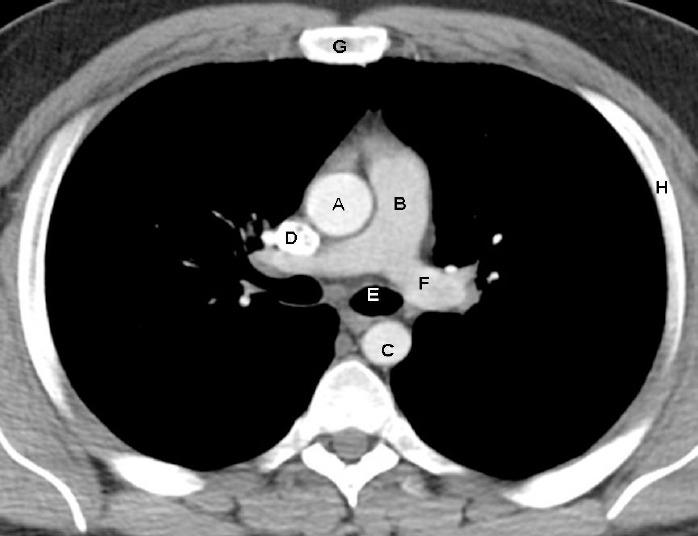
On post contrast enhanced axial CT image of the chest of a 40 years old man shown above, identify the labelled normal anatomical structures
